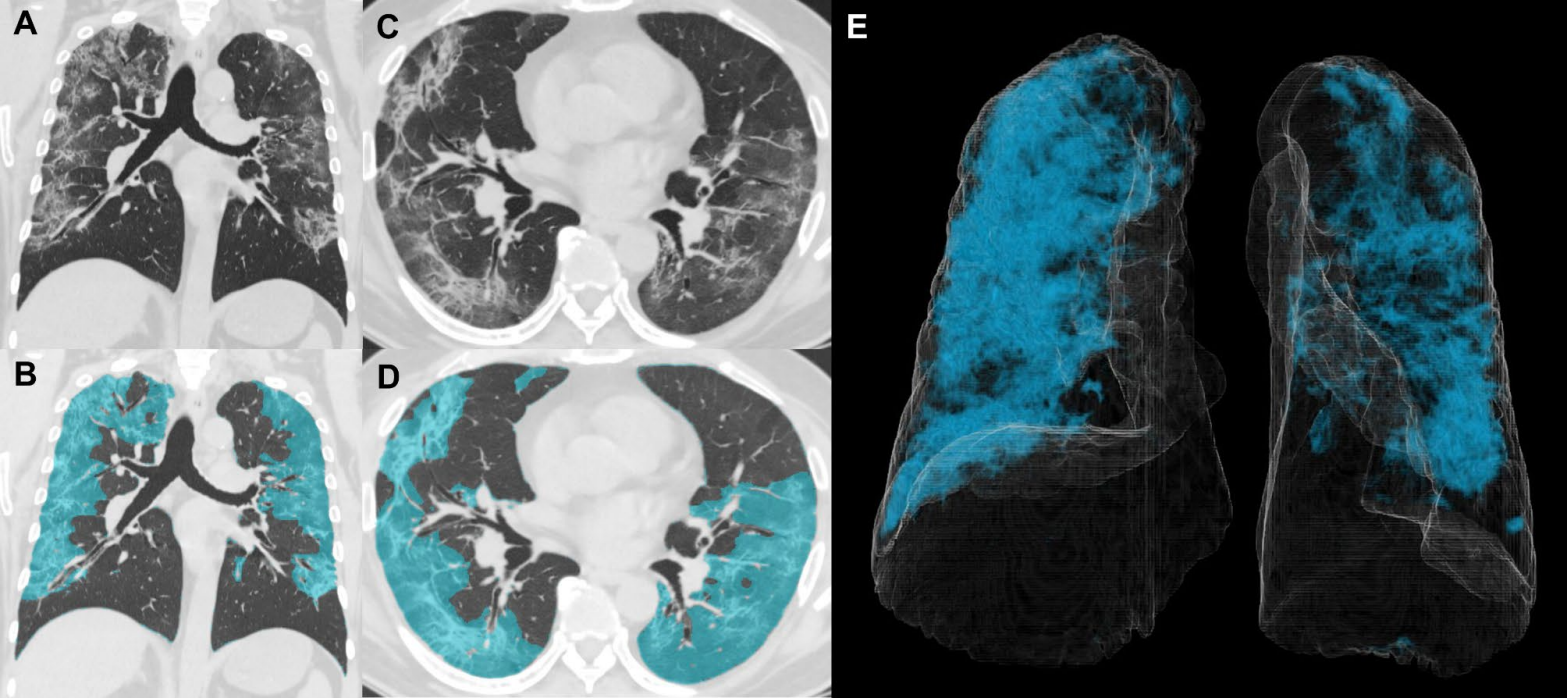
Image of one patient with COVID-19 showing segmentation of the entire lung and pneumonia. (A-D) Chest CT images of severe COVID-19 cases (A: axial, C: coronal) and segmentation of pneumonia lesions (highlighted in Blue) by AI-based analysis (B: axial, D: coronal). (E) Segmentation of the total lung (White curved surface) and pneumonia (Blue highlighted) using 3D viewer images. In this representative case, the total lung volume was 3890 mL, the volume of lung lesions was 1314 mL, and the percentage of lung lesions was 33.8%
